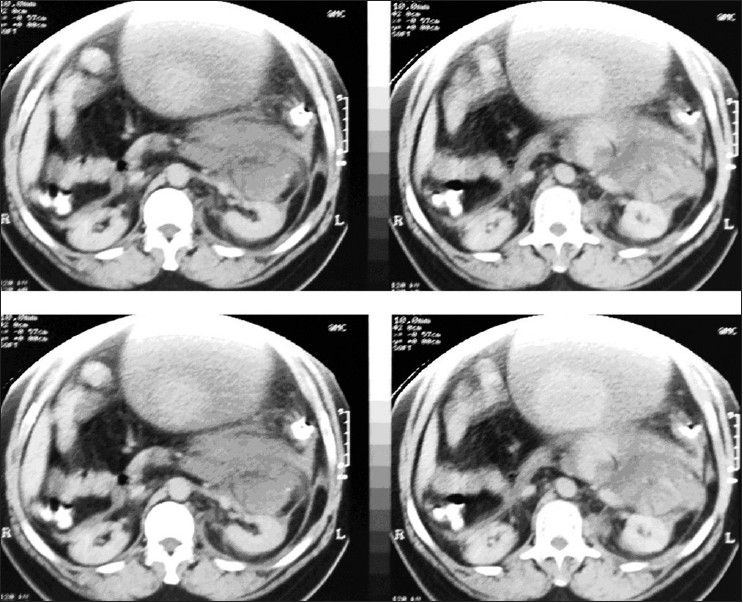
CT scan shows huge multi-locular blood cyst filling most of the abdominal cavity
